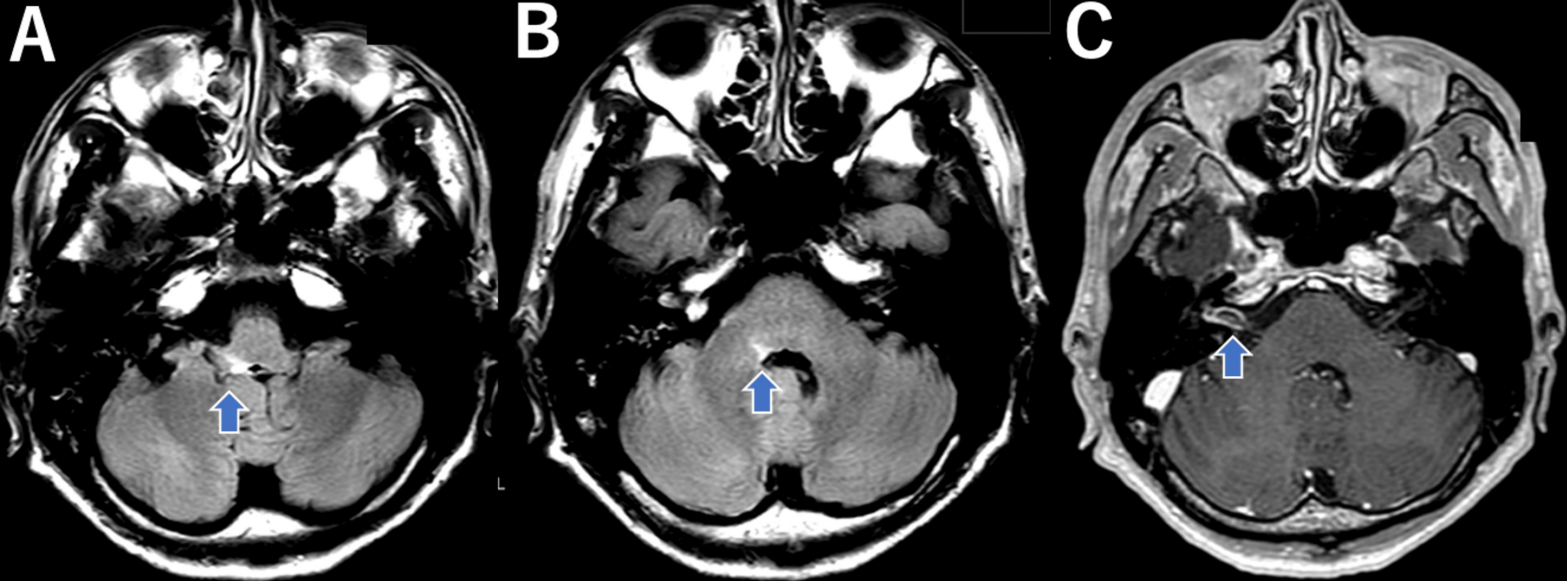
Head MRI, FLAIR sequence, showed high signal intensity in the right vestibular nuclei and surrounding areas. On contrast-enhanced MRI, enlargement of the facial nerve was observed.
